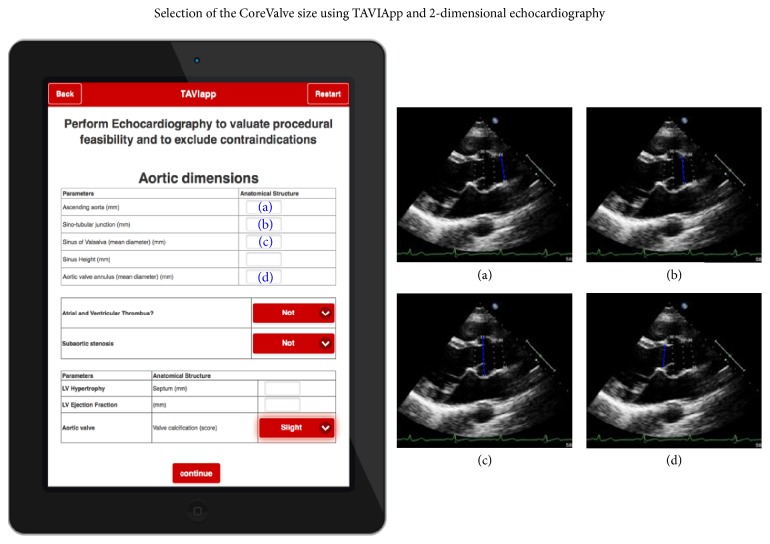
Selection of prosthesis size with TAVIApp using 2D echocardiography results. The figure reports a screenshot from TAVIApp where the user can input two-dimensional echocardiography measurements. When a CT scan is not available, TAVIApp selects the most appropriate CoreValve size using the following 2D echocardiography data: (a) diameter of the ascending aorta; (b) aortic diameter at the sinotubular junction; (c) aortic diameter at valsalva sinuses; (d) mean diameter of the aortic valve annulus.
